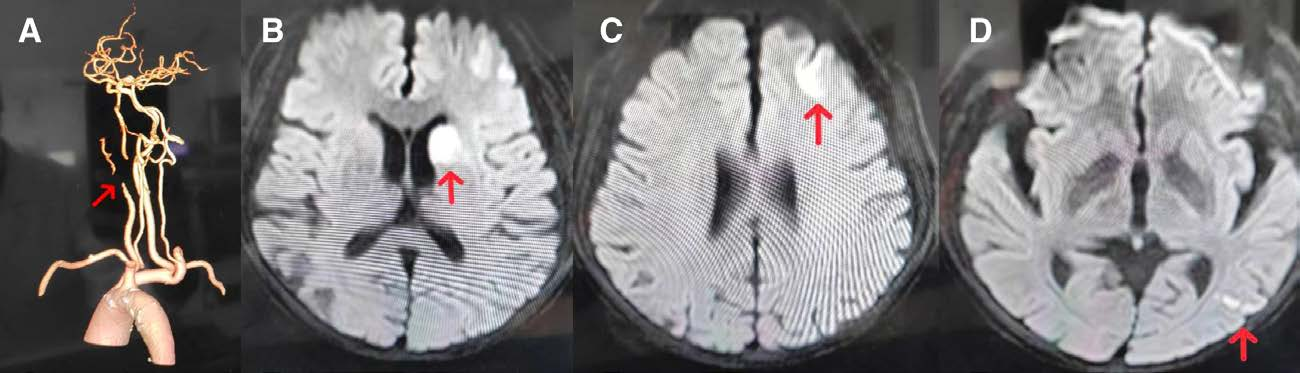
(A) Head and neck CTA demonstrated stenosis in the C1 segment of the left internal carotid artery, with the site of narrowing indicated by the red arrow. (B–D) Cranial MRI showing acute cerebral infarcts in the bilateral frontal lobes, left temporo-parieto-occipital region, and basal ganglia. The red arrow indicates the site of corresponding vascular stenosis. CTA = computed tomography angiography, MRI = magnetic resonance imaging.
